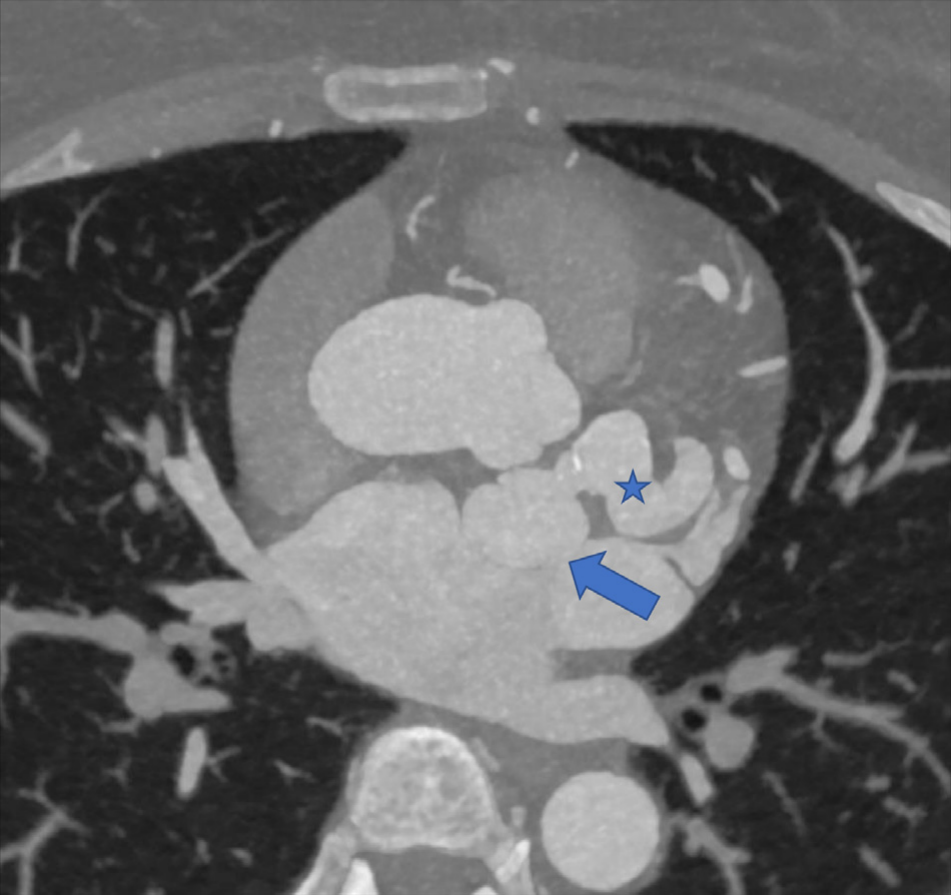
Axial contrast-enhanced CTA maximum intensity projection image: the distal portion of the LCX CAF (blue star) connects to the left atrium (blue arrow) near the left atrial appendage.
